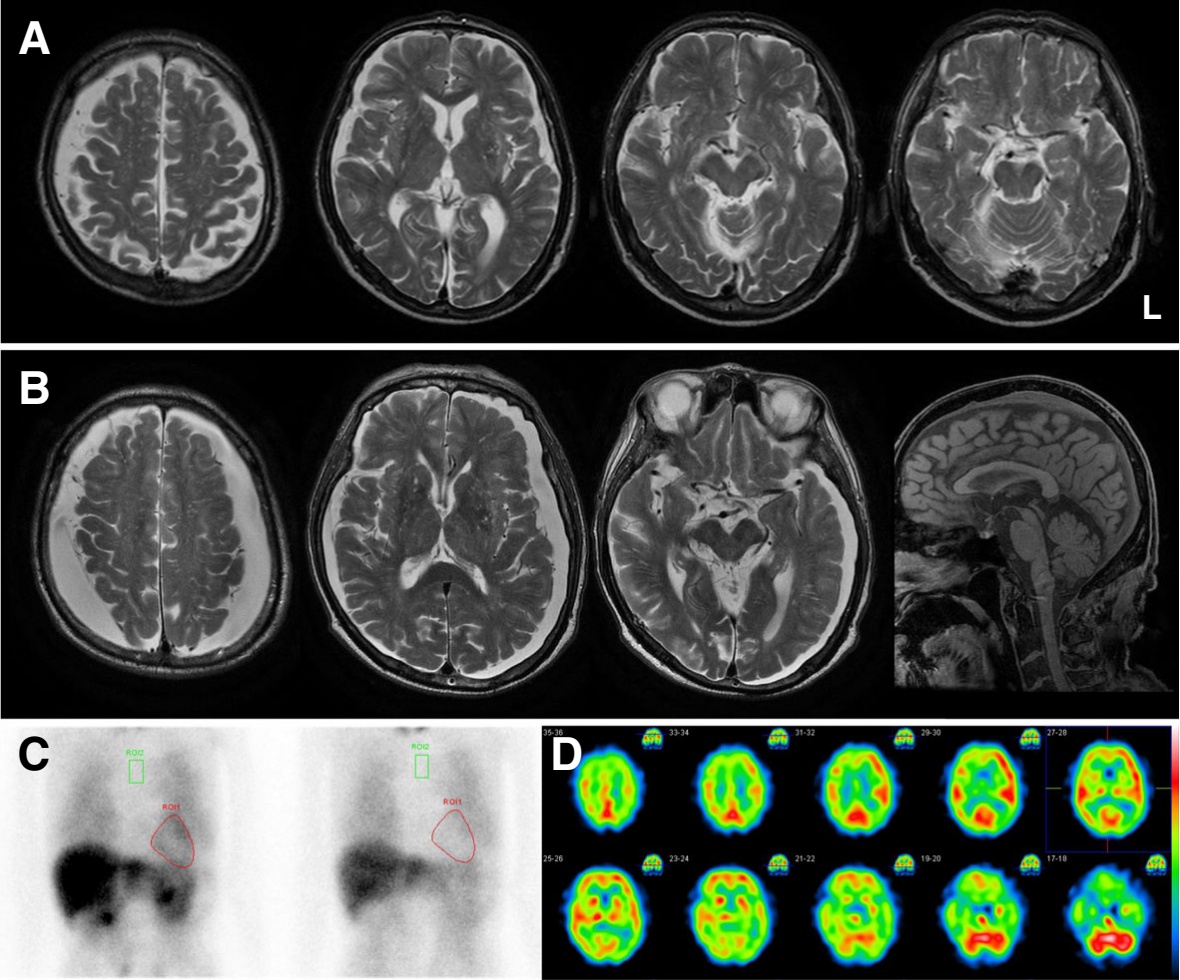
Imaging findings. a A brain MRI performed at 77 years old revealed mild frontal lobe atrophy and mild right-dominant subdural hygroma. b A follow-up MRI performed at 79 years old showed increased subdural hygroma and atrophy in the midbrain tegmentum. c Cardiac ^123^I-meta-iodobenzylguanidine scintigraphy showed decreased uptake in the delayed phase, suggesting mild sympathetic denervation. d Brain N-isopropyl-p-[^123^I]iodoamphetamine scintigraphy showed hypoperfusion in the bilateral frontal, parietal, and occipital lobes but not the basal ganglia, thalamus, and cerebellum
